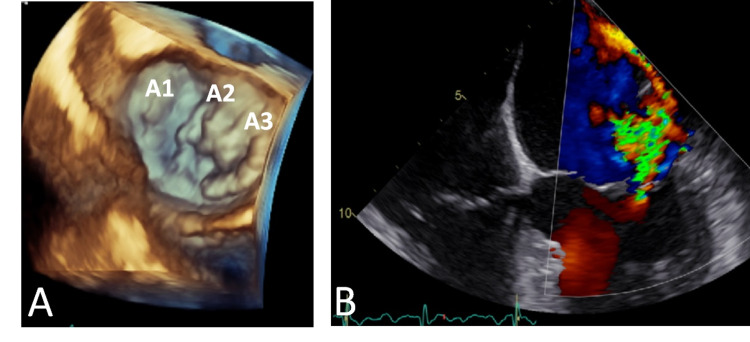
Transesophageal echocardiography showing a severe mitral regurgitation, due to anterior leaflet prolapse at A2 and A3 scallops. The eccentric jet, directed laterally and posteriorly, reaches the left atrium's ceiling. A) Three-dimensional imaging of the mitral valve, atrial view; B) mid-esophageal 4-chamber view (0º) with colour Doppler.
